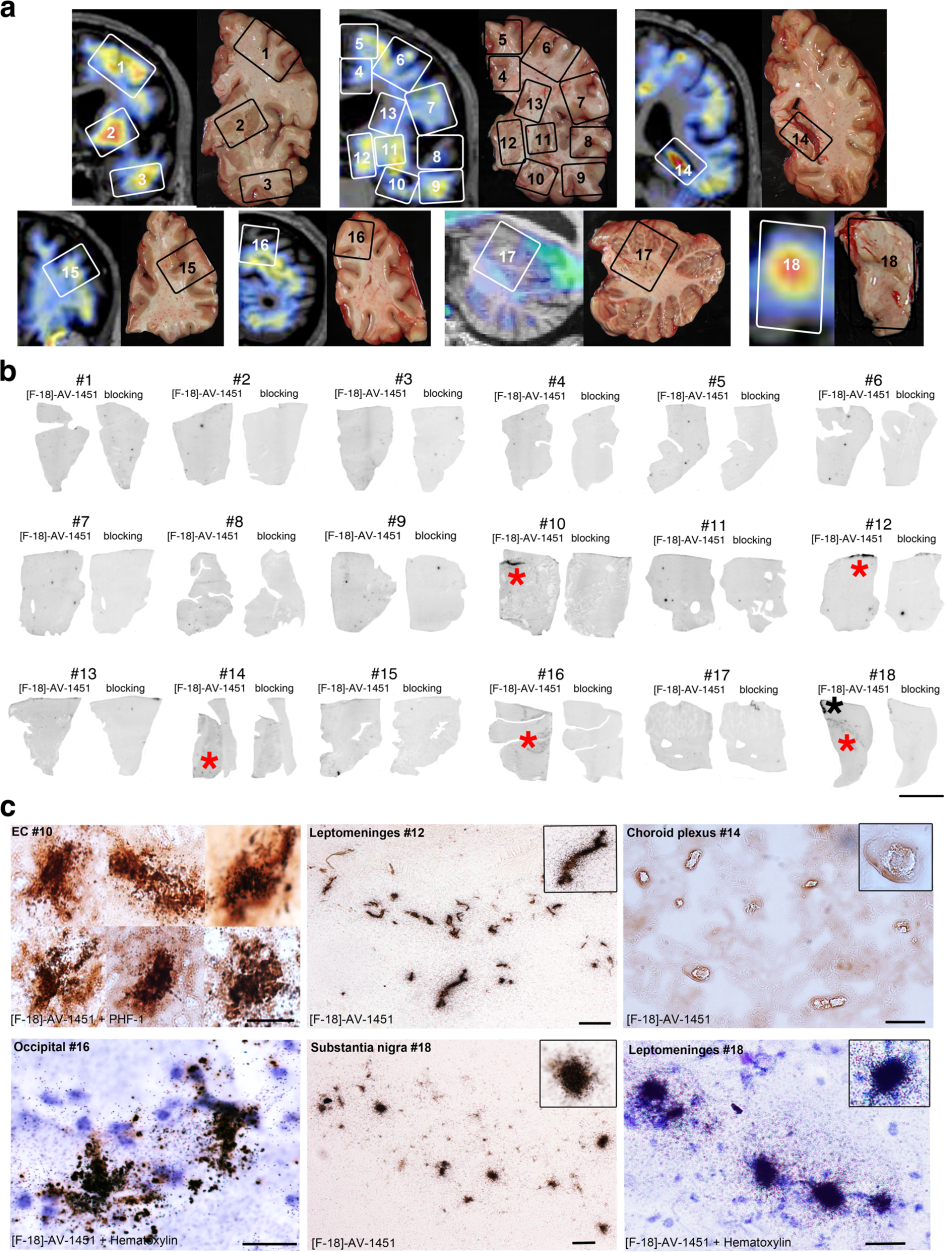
Coronal in vivo [F-18]-AV-1451 PET images superimposed to brain MRI (a, left), matching autopsy tissue blocks (a, right), phosphor screen autoradiography (b), and microphotographs of nuclear emulsion dipped slides after incubation with [F-18]-AV-1451 (c) from the PD subject. The numbers displayed on PET and autoradiography images correspond to matching ROIs. Most ROIs analyzed did not exhibit detectable autoradiography signal, with the exception of the EC (red asterisk) (#10, reflecting Braak II age-related NFT pathology), leptomeningeal melanocytes adjacent to the lateral ventricle (red asterisk) (#12), and the mesencephalus (red and black asterisks) (#18, reflecting *off-target* binding to neuromelanin-containing neurons in the substantia nigra and meningeal melanocytes, respectively). The choroid plexus (red asterisk) (#14) displayed a very faint autoradiography signal that was not blocked with 1 μM unlabeled AV-1451. Numbers correspond to the following anatomical regions: #1 = middle frontal, #2 = putamen and pallidum, #3 = inferior temporal, #4 = anterior cingulate, #5 = superior frontal, #6 = middle frontal, #7 = inferior frontal, #8 = superior temporal, #9 = inferior temporal, #10 = HPC/EC, #11 = putamen and pallidum, #12 = thalamus, #13 = caudate, #14 = choroid plexus, #15 = middle frontal, #16 = occipital, #17 = cerebellar cortex and dentate nucleus, and #18 = substantia nigra. Abbreviations: EC = entorhinal cortex, HPC = hippocampus; MRI = magnetic resonance imaging; NFT = neurofibrillary tangle; PD = Parkinson’s disease; PET = positron emission tomography; ROI = region of interest. Scale bar = 1 cm (b), 20 μm (c)
